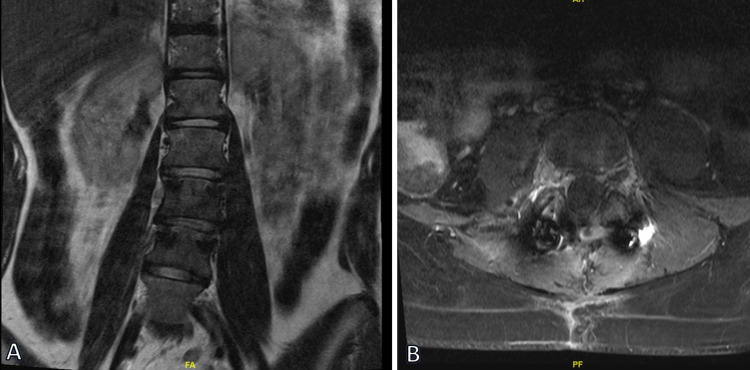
Postoperative MRI demonstrating near-complete excision and fusion of L2-L5. (A) T2-weighted coronal and (B) T1-weighted postcontrast axial MRIs.
